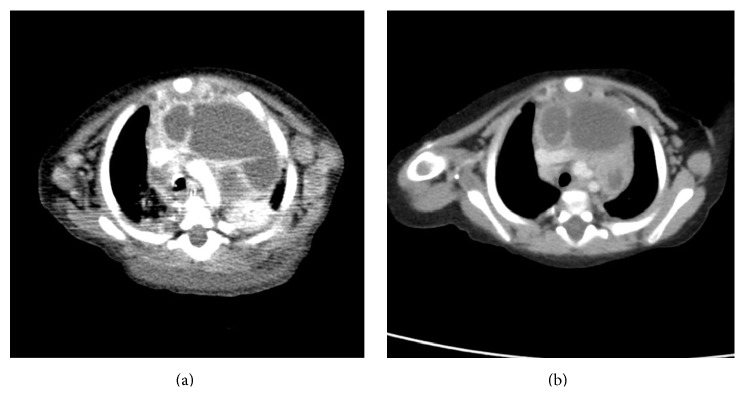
CT scan of chest showing reduction of mediastinal lesions.
